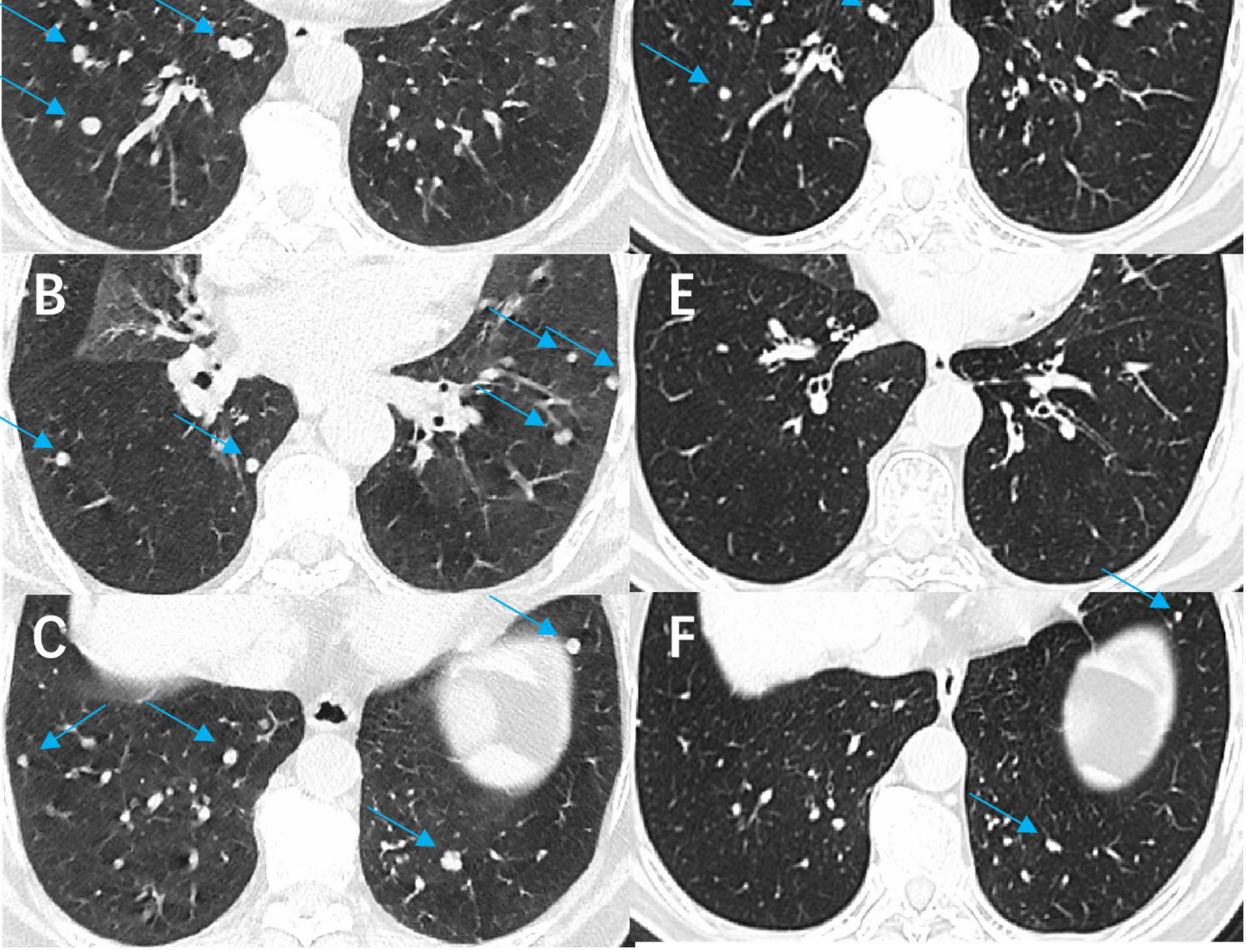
Comparison of images before and after treatment with anlotinib combined with ^125^I seed implantation in a patient with refractory follicular thyroid carcinoma. The patient had been taking anlotinib since March 2022. (A-C) show the CT images of both lungs before treatment (arrows indicate the lesions), and (D-F) show the corresponding CT images after treatment. A significant reduction in the number and size of pulmonary metastatic lesions is evident.
